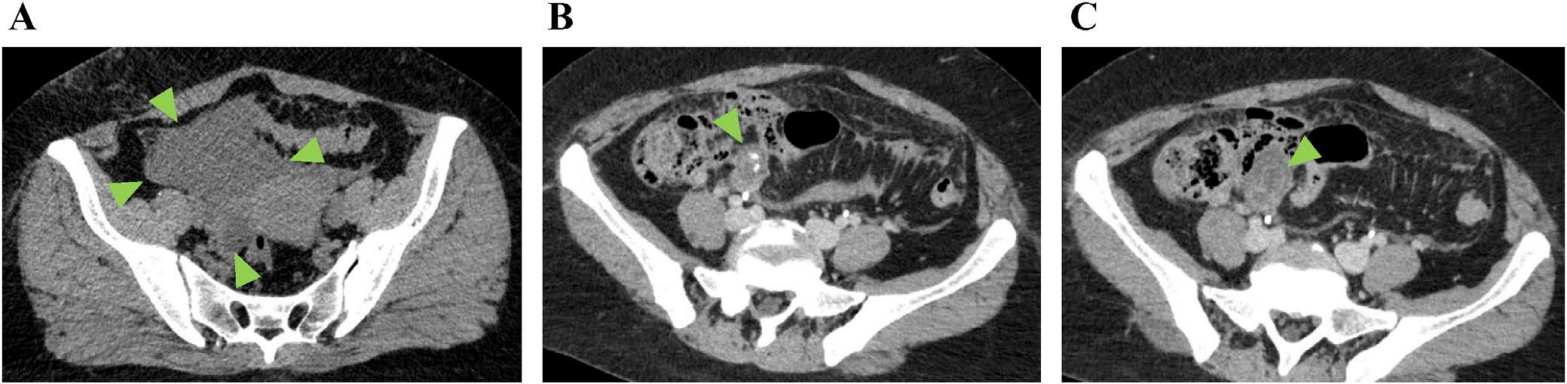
Abdominal computed tomography at presentation. (A) Plane image showing a peritoneal fluid around the Douglas fossa extending to the ileocecal area with a mean Hounsfield units of 28 (triangle arrow), indicating complex fluid due to intra‐abdominal injury. (B) Contrast‐enhanced image highlighting the extended tip of the appendix with a coprolith (triangle arrow). (C) Contrast‐enhanced image demonstrating an unclear appendiceal wall structure at the tip of the appendix (triangle arrow).
